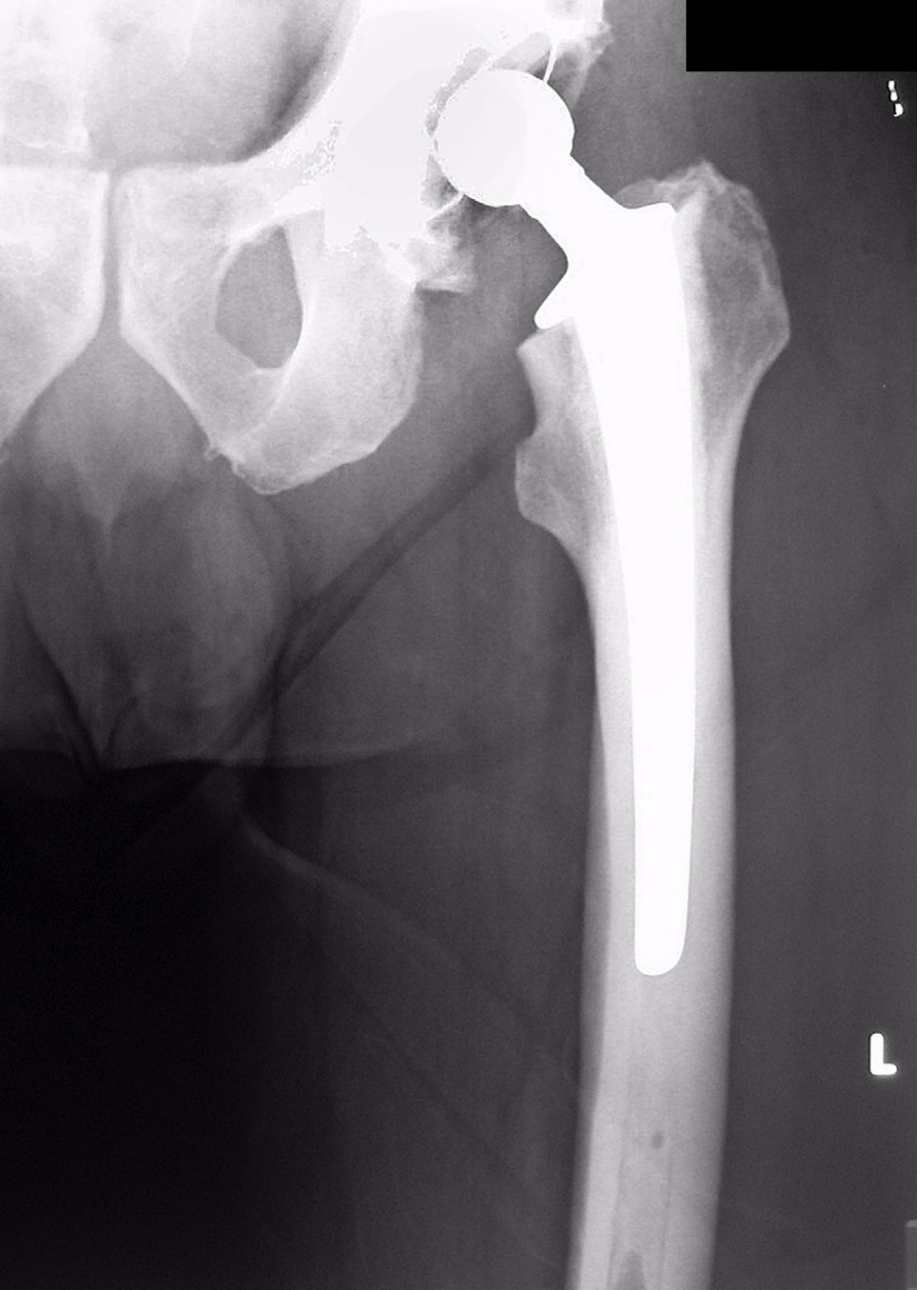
Postoperative radiograph of the left hip at 5 days showing the total hip replacement.
